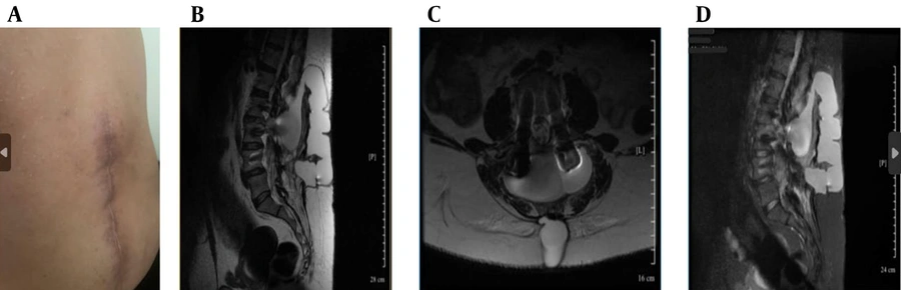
Clinical and magnetic resonance imaging (MRI) findings (A - D): A, postoperative lumbar scar with signs of cerebrospinal fluid (CSF) leak; B, sagittal T2-weighted MRI showing posterior epidural CSF collection; C, axial T2-weighted MRI showing hyperintense fluid posterior to the thecal sac; D, sagittal short tau inversion recovery (STIR) MRI showing cranially extending epidural CSF leak.
